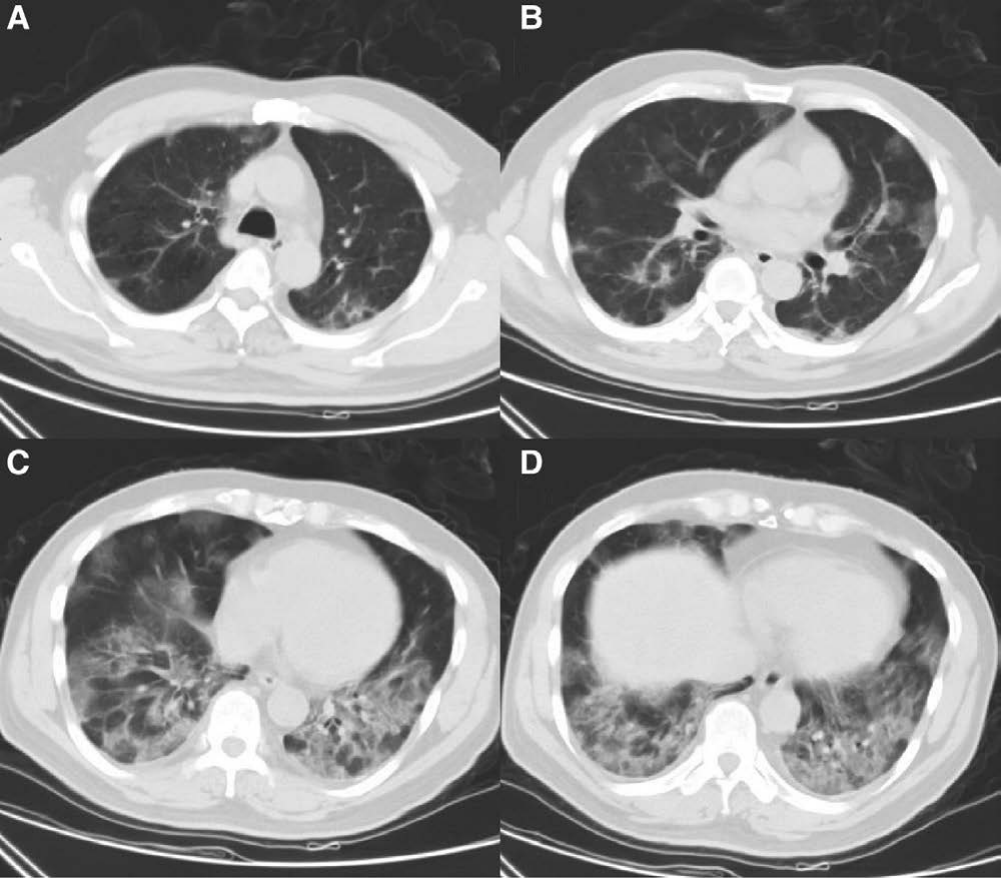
The initial chest CT scan of the patient on the 1th day of admission (A–D): showed multiple “ground glass” opacities in both lungs. CT = computed tomography.
